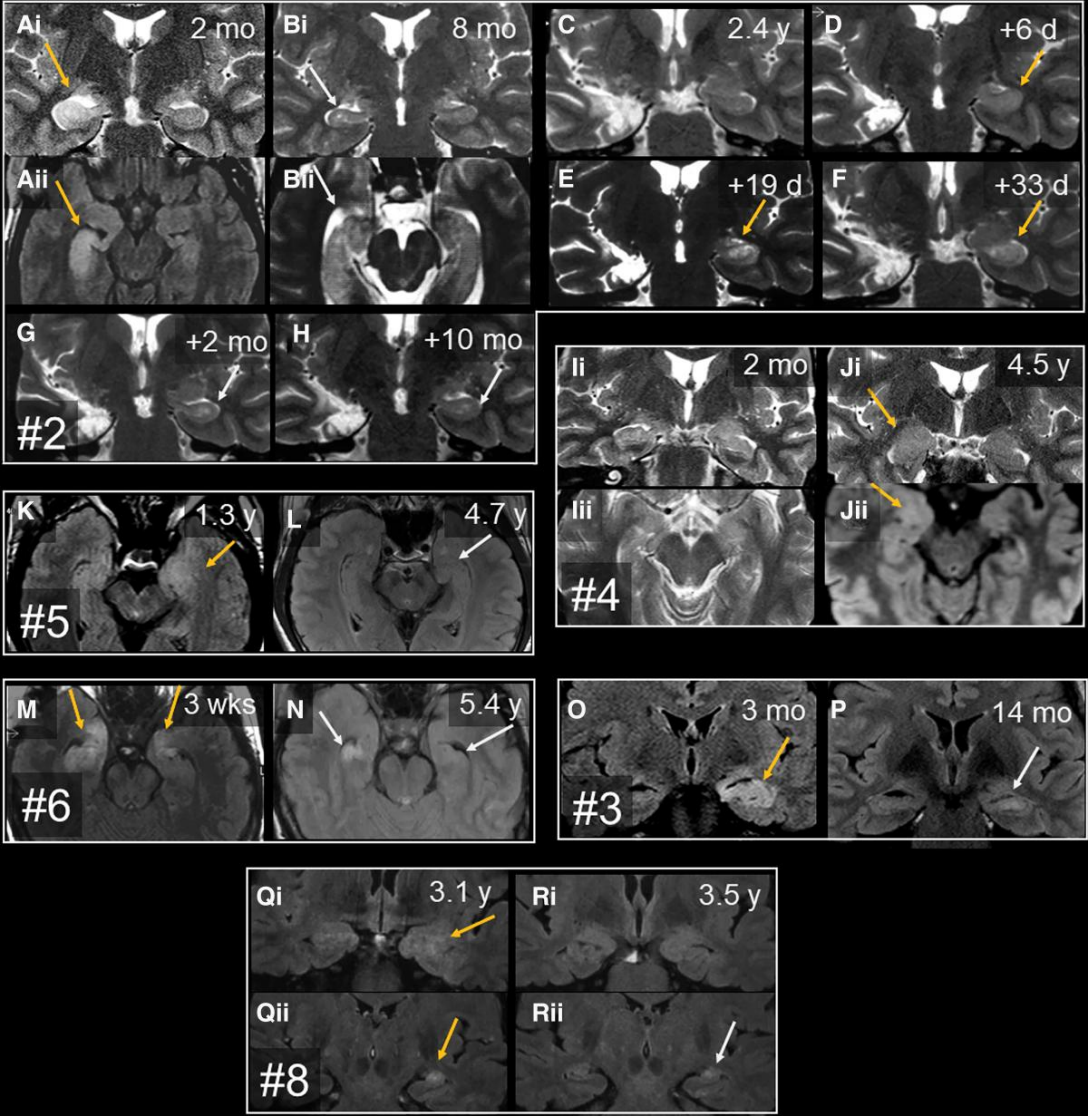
Serial T_2_ or FLAIR MRI with time since disease manifestation. The images show the mediotemporal lobes with swelling and signal increase (orange arrows), HS (grey arrows) or normal findings. Each box contains images from one patient. Each letter marks one study time point; subscript numbers discriminate different orientations or planes. (A–H) Patient 2. [A(i and ii)] Two months, right-sided hippocampal volume and signal increase, amygdala unaffected. [B(i and ii)] Six months later, there is right-sided HS and still no amygdalar lesion. (C) Almost 2 years after right-sided selective amygdalohippocampectomy and start of prednisolone therapy, the patient is seizure-free and still has intact left mediotemporal structures. Prednisolone was discontinued. (D) A few days later, the patient developed left temporal status epilepticus, and the left hippocampus showed increased volume and T_2_ signal. (F and G) Close-meshed follow-up MRIs over the following 2 months revealed how this resulted in left HS. (H) Final stage. (I and J) Patient 4. [I(i and ii)] Two months after epilepsy onset, mediotemporal structures were normal; 3.8 years after onset, there was swelling and signal increase of the right amygdala (not shown). [J(i and ii)] These changes were still present 9 months later, that is, 4.5 years after onset. In this case, the amygdala but not the hippocampus was affected; immediately after J, the right amygdala was resected because a glioma was suspected. (K and L) Patient 5. (K) The first available MRI at 1.3 years after onset displays the left amygdalar and hippocampal volume and signal increase. (L) On the next available MRI study, there is already hippocampal atrophy (better visible on the not shown coronal sections). (M and N) Patient 6. (M) Three weeks after onset, there is bilateral swelling and signal increase of both hippocampal heads and amygdalae. (N) Five years later, the MRI reveals bilateral HS. (O and P) Patient 3. (O) At 3 months, the left hippocampus (and amygdala, not shown) are swollen and hyperintense. (P) Fourteen months after onset, left-sided HS has evolved. (Q and R) Patient 8. [Q(i and ii)] On the earliest available images taken 3.1 years after onset, the left amygdala and hippocampus are swollen and hyperintense. [R(i and ii)] Four months later, the left amygdala has returned to normal [R(i)], while the hippocampus has become atrophic with still increased signal, that is, HS [R(ii)]. d = days; mo = months; wks = weeks; y = years.
